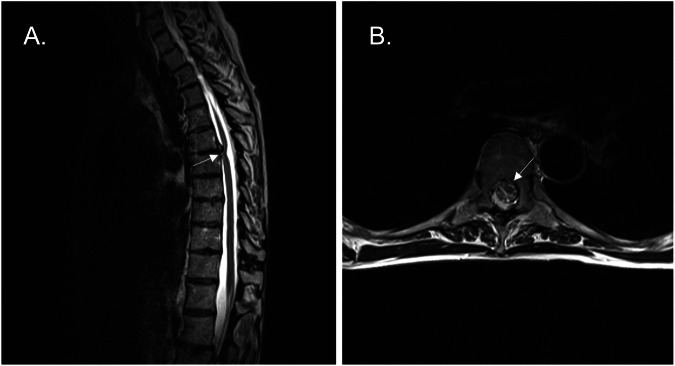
MRI featuring ISCH. A Sagittal and B axial MRI demonstrate sharp anterior angulation or the thoracic spinal cord with absence of CSF ventrally and widening of the subarachnoid space dorsally.
